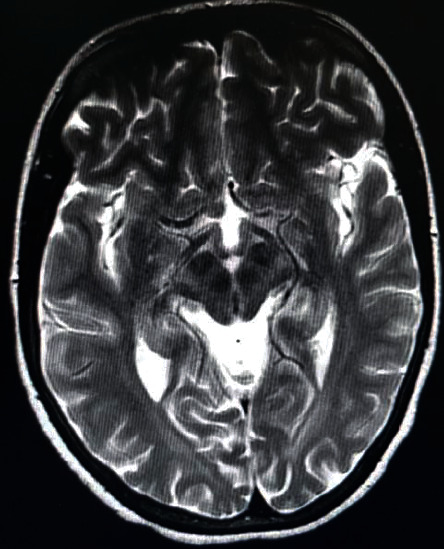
Axial T_2_-weighted MRI image showing classic “face of the giant panda” sign in midbrain hyperintensity of the tegmentum except for the red nucleus (the eyes), substantia nigra (the ears), and superior colliculus (the mouth) (case-2).
